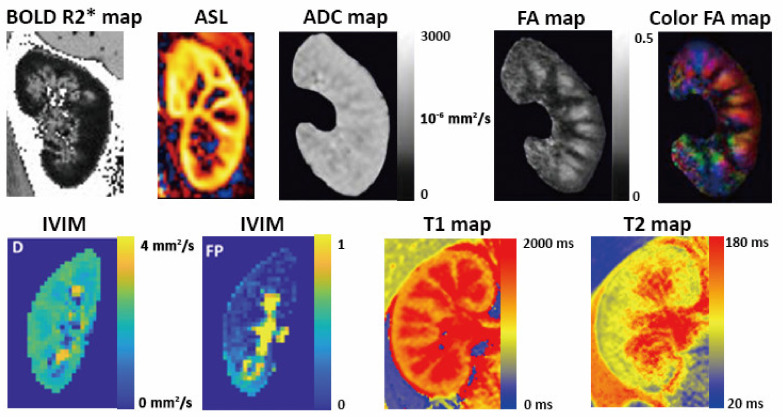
Multiparametric kidney MRI in healthy volunteers. BOLD R2* map is used to evaluate parenchyma oxygenation. Renal blood flow can be quantified from ASL perfusion weighted image. ADC and FA maps generated from DWI and DTI can be used to assess renal fibrosis. IVIM imaging evaluates true parenchyma diffusion by separate modeling. Renal T1 maps showing clear cortico-medullary differentiation in a healthy volunteer and T2 mapping are promising techniques to evaluate renal microstructure. The BOLD R2* map, ADC map, FA maps and IVIM images were adapted from the articles of Bane et al. [71], Adams et al. [76], and de Boer et al. [77] under Creative Commons licenses.
